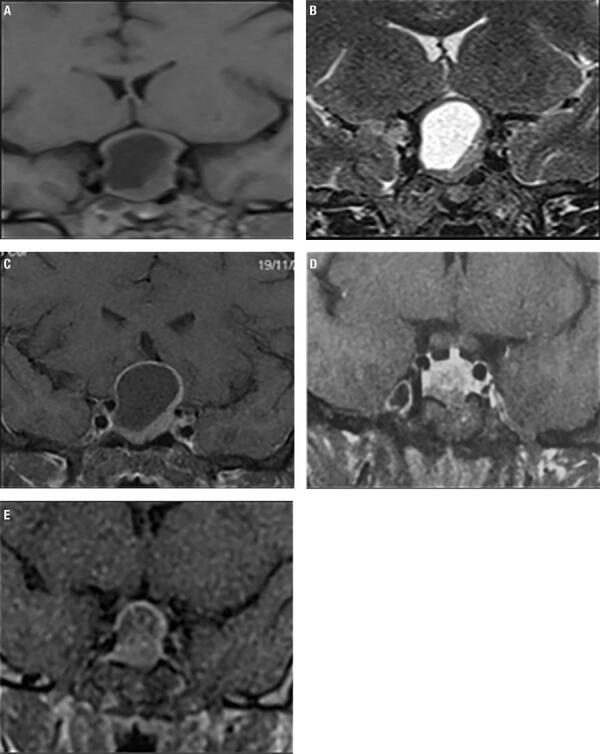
Case 1 Pituitary MRI without gadolinium during pregnancy (A-B) and postpartum/postsurgery MRI with gadolinium (C-D-E): Coronal view of a large pituitary mass, hypointense on T1-weighted images (A), and hyperintense on T2-weighted images, with suprasellar extension and compression of the optic chiasm (B). Coronal view of a stable post-partum image after gadolinium-enhancement (C). Post-surgery pituitary MRI with gadolinium: coronal view of a small tumor remnant, T1-weighted images (D). Coronal T1-weighted image shows tumor re-expansion after 4 years of surgery (E).
